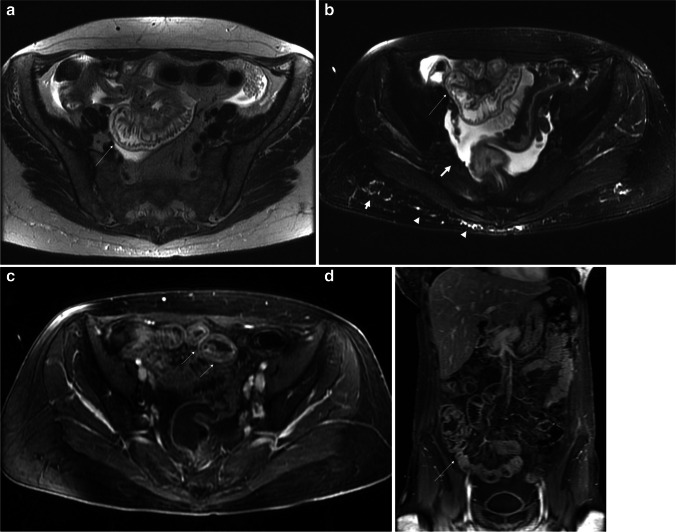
Acute intestinal GVHD in a 40-year-old woman, Glucksberg grade I (low clinical grade). The calculated MRI severity score is 12, considered as a moderate-grade disease (low mortality risk). Only the ileum is involved. a (T2-weighted axial image) and b (T2-weighted fat-suppressed axial image) show mild thickening with *edema and stratification* of the bowel wall (thin arrows); in addition, in the fat-suppressed image, a slight *ascites* and mild *edema of the declivous subcutaneous* (arrowheads) can be observed. c (T1-weighted gadolinium-enhanced axial image) and d (T1-weighted gadolinium-enhanced coronal image) show post-contrast enhancement and stratification at the level of the affected bowel (thin arrows)
